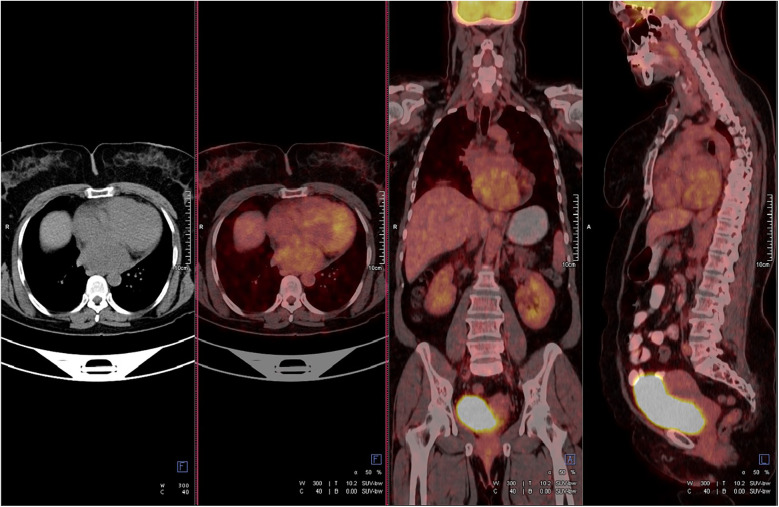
Positron emission tomography-computed tomography showed mild to moderate fluorodeoxyglucose uptake in the mass. The standard uptake value was ∼5.2. No metastatic lesions were detected.
